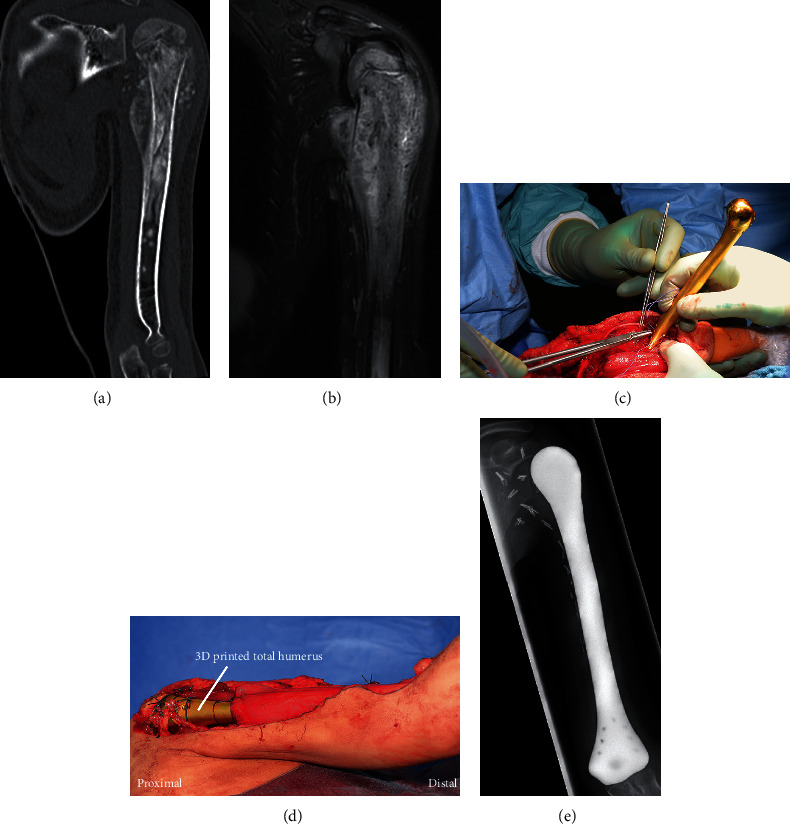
A five-year-old girl with a high-grade osteosarcoma of the left proximal humerus. (a, b) CT and MRI scans showed a locally advanced tumor involving almost the complete humerus. (c, d) Reconstruction of the osseous defect by the 3D printed total humerus. (e) X-ray image one year after surgery showed no dislocation.
